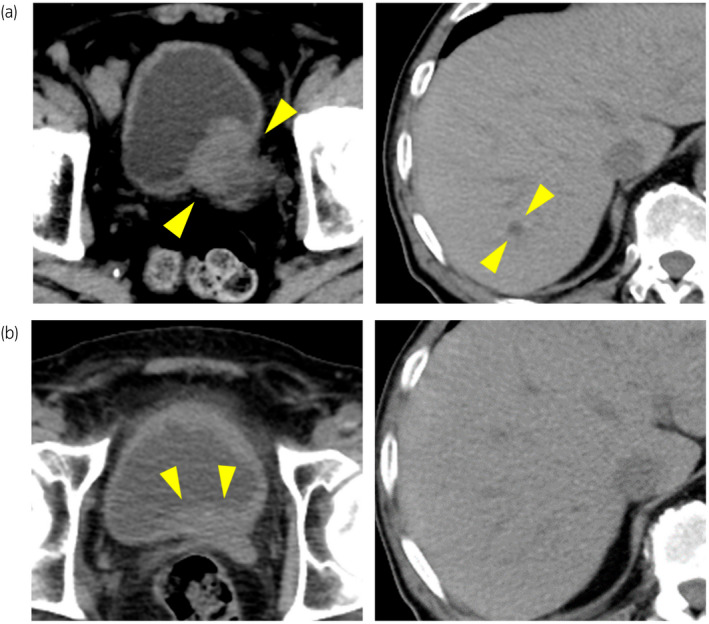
(a) CT at the first visit. A 43‐mm‐sized mass was detected on the left posterior wall of the bladder, accompanied by a twitch in the wall, and an 8‐mm‐sized low‐concentration nodule in size in the S6 region of the liver. (b) CT after irinotecan and carboplatin treatment. The bladder tumor shrank significantly, with only a mild thickening of the bladder wall. The liver tumor was obscured.
